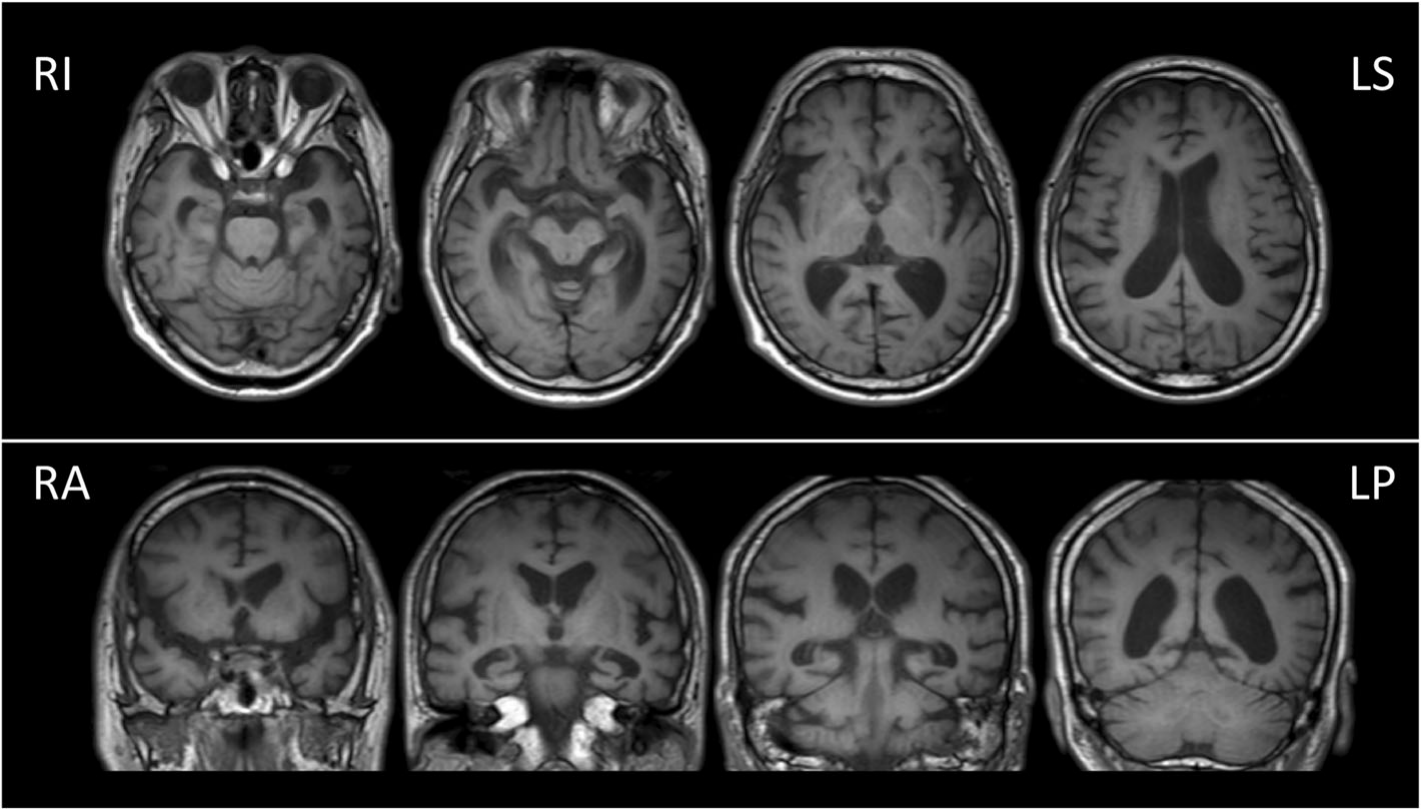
Brain magnetic resonance imaging. Brain magnetic resonance imaging (MRI) showing predominantly left-sided atrophy of the anterior and medial temporal lobe and enlargement of the left lateral ventricle. LP, left posterior; LS, left superior; RA, right anterior; RI, right inferior
